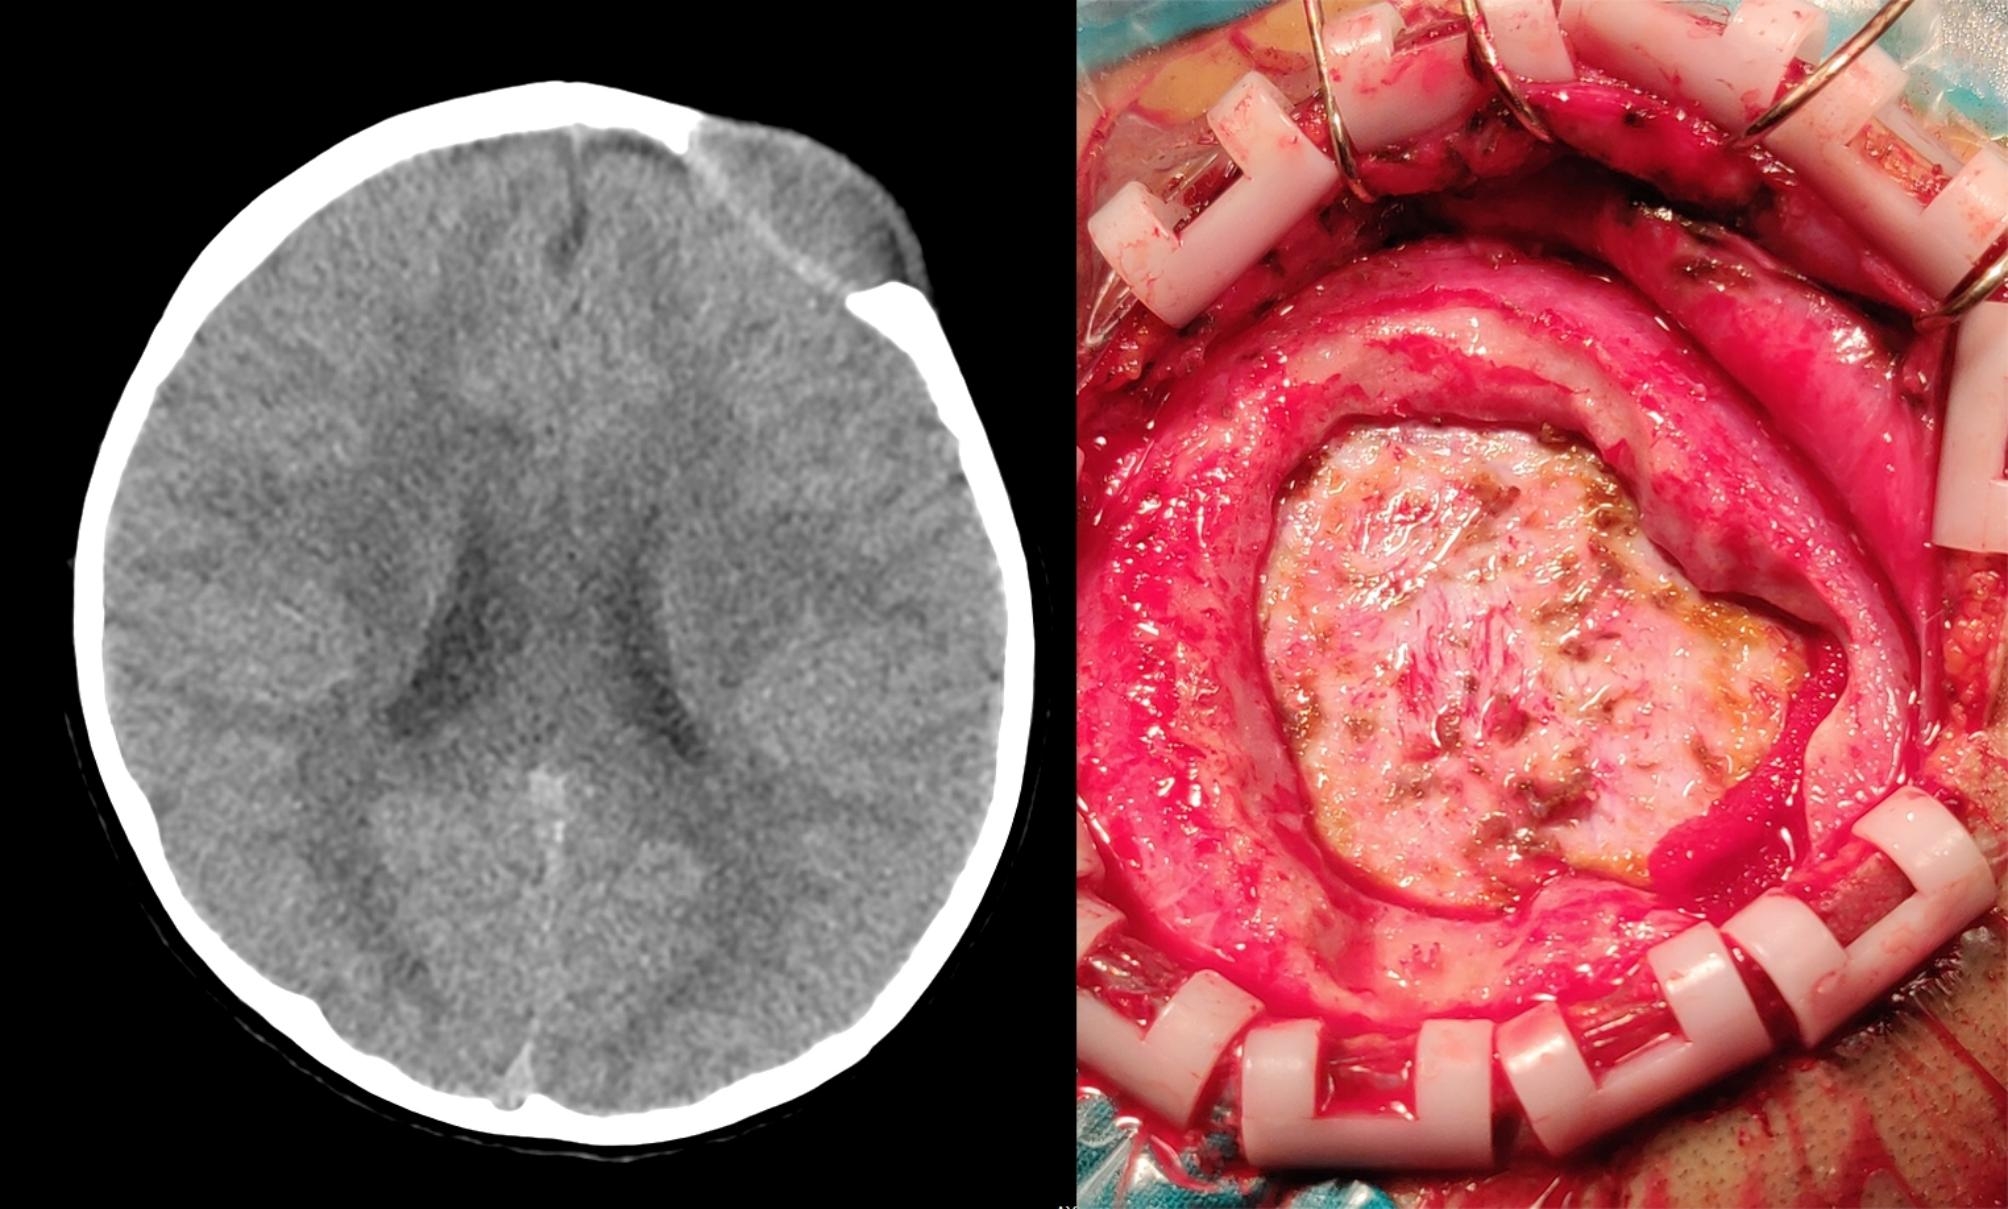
The Rosai-Dorfman disease CT and surgical picture, the CT (left) showed the fusiform soft tissue lesions are located in frontal subcutaneously continue to the skull, forming a large 5*5 cm defect on skull, and the surgery picture (right) showed a large skull defect was revealed after the mass was removed
